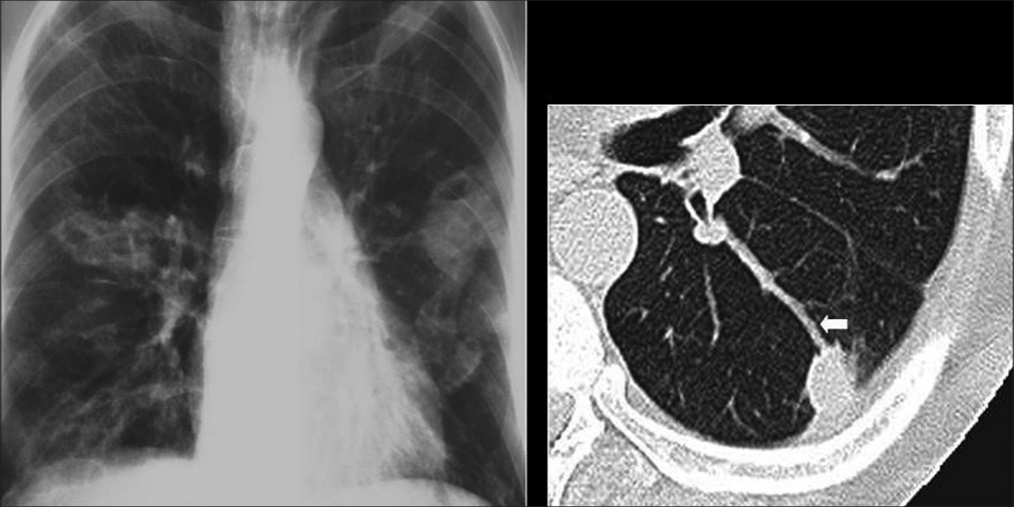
Septic emboli. A chest X-ray (CXR) and high-resolution computed tomography (HRCT) on a human immunodeficiency virus-positive patient with gram negative septicemia and septic emboli. Note the wedge-shaped pleural-based opacity and the feeding vessel on HRCT (arrow) and multiple cavitating nodules on the CXR
